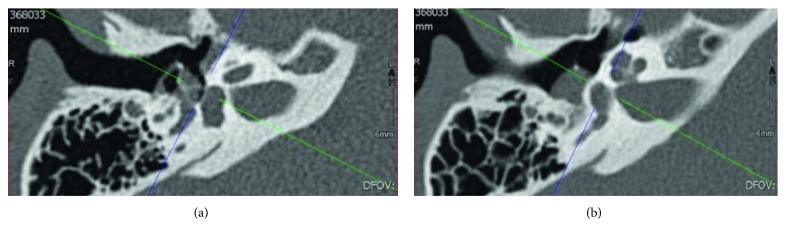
2008 high-resolution multidetector computed tomography (MDCT) (axial views) showing nonosteolytic nodular tissue mass in the hypotympanum alongside the promontory.
